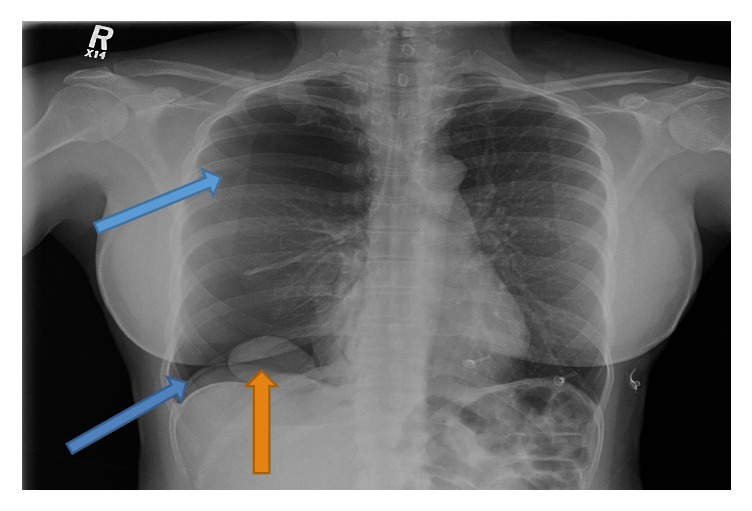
The blue arrows show the visceral pleural line indicating the presence of pneumothorax and diaphragmatic free air. The orange arrow reveals a large 56 mm mass at the base of the lung (confirmed as liver on computed tomography).
